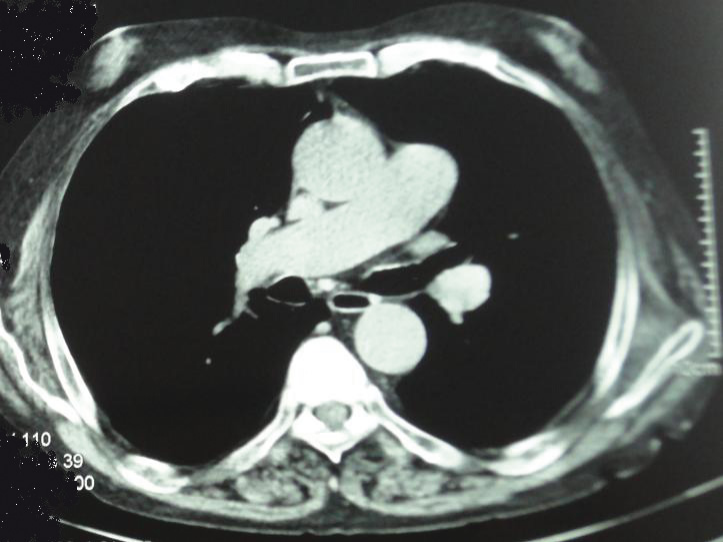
Lumina of air bubble going down thorax in previous patient as in Figure 1. This series of CT scans shows landmarks for distance down esophagus.
